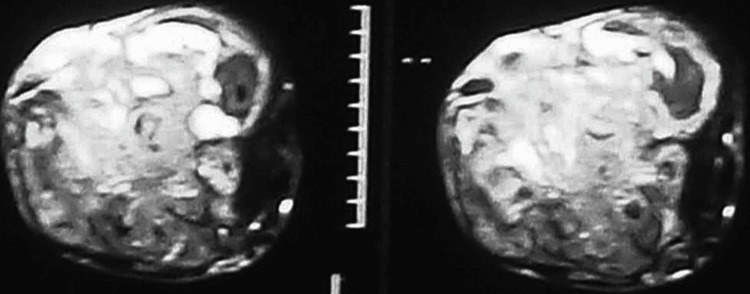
MRI image of the right elbow showing entrapment of the radial nerve in the substance of the tumor
